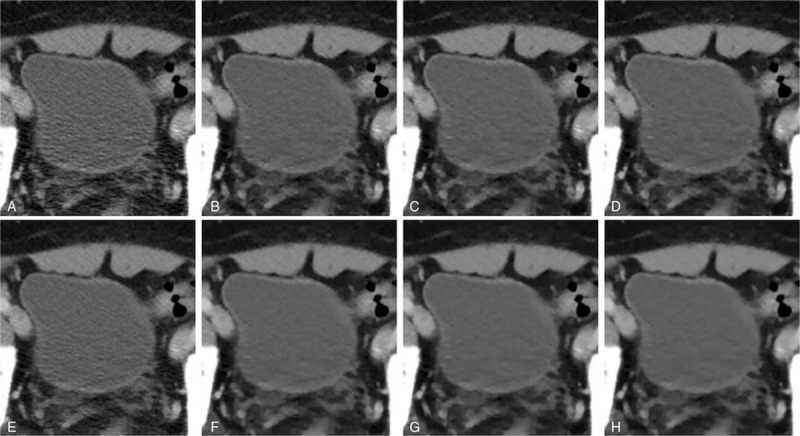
Comparison of abdominal computed tomography image quality focusing on the urinary bladder of a 63-year-old male patient. (A) FBP; (B) IMR-R-L1; (C) IMR-R-L2; (D) IMR-R-L3; (E) iDose4; (F) IMR-ST-L1; (G) IMR-ST-L2; and (H) IMR-ST-L3. Noise granules were evident in the FBP (A) and iDose4 (E) reconstruction algorithms. Noise was reduced after applying IMR (B–H), yielding images that were more homogenous. FBP = filtered back projection, IMR = iterative model reconstruction, L = level, R = routine, ST = soft tissue.
